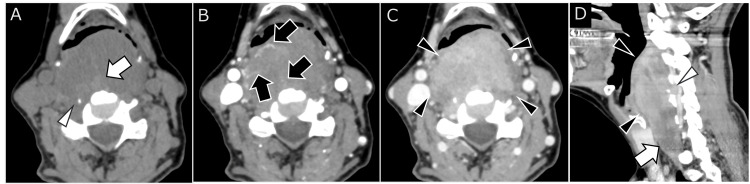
Non-contrast and contrast-enhanced CT images. A soft-tissue mass extends from the retropharyngeal to the danger space at the oropharynx to the thyroid level.
